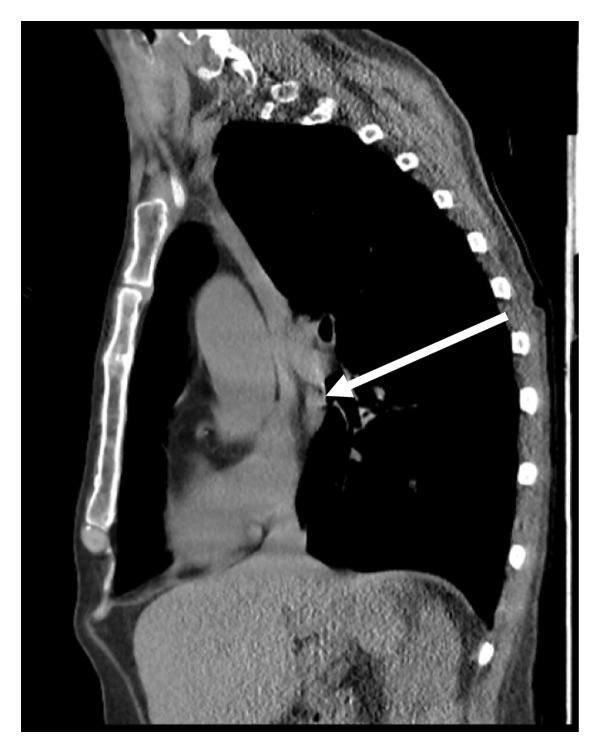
Previous (2019) sagittal CT showing the retroaortic PLSVC path. The PLSVC can be seen extending much further inferiorly than would be expected of an internal jugular vein (white arrow).
